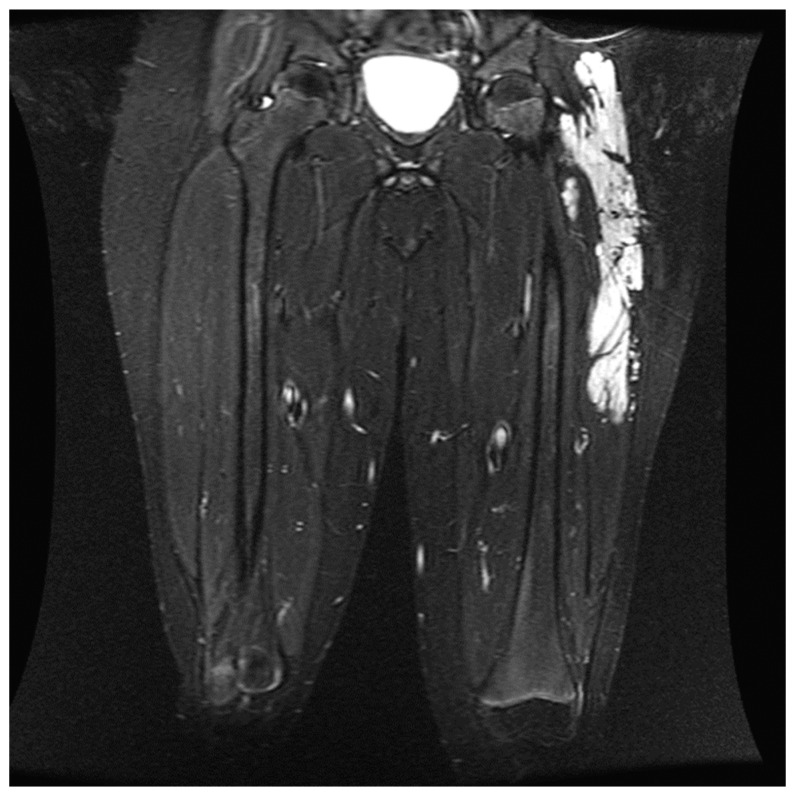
Coronal T2-weighted fat-suppressed image shows an extensive hyperintense slow flow vascular malformation consistent with a venous malformation involving the intramuscular and subcutaneous planes of the left lateral thigh and hip. During the specified range of dates, the child received four procedures. Each procedure was performed with a lumbar plexus nerve block for postoperative pain control.
